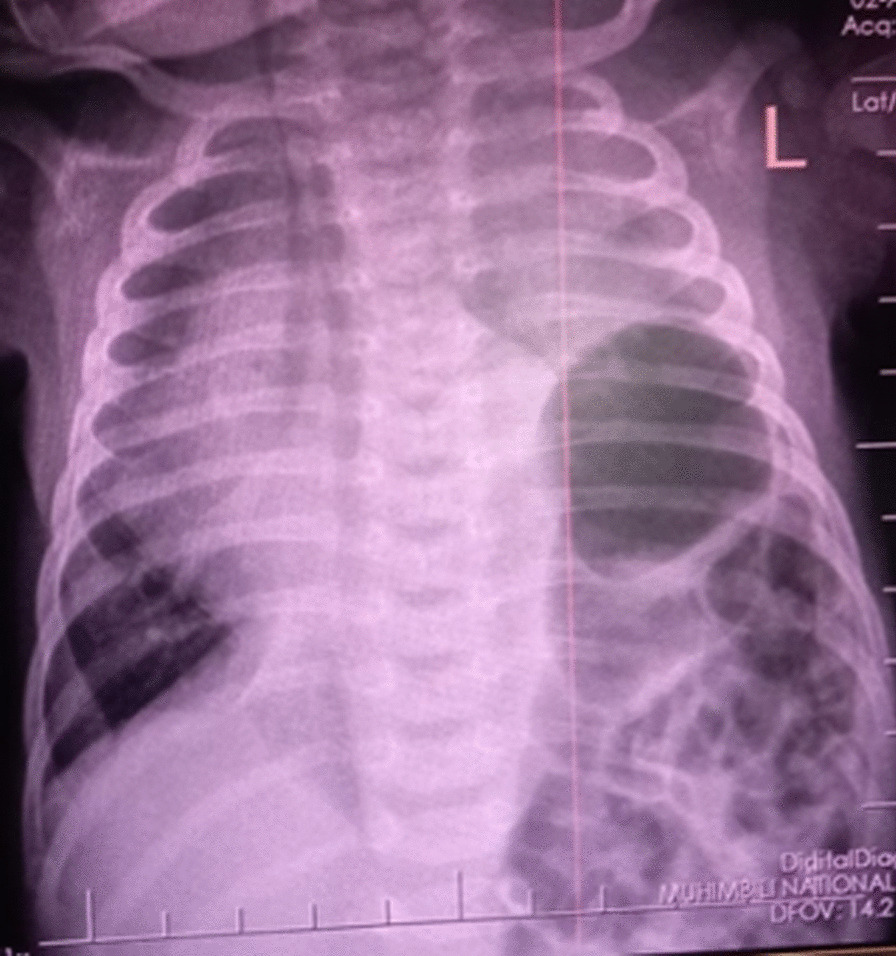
Chest X-ray showing herniated bowel loops into the left hemithorax with mediastinal right shift
